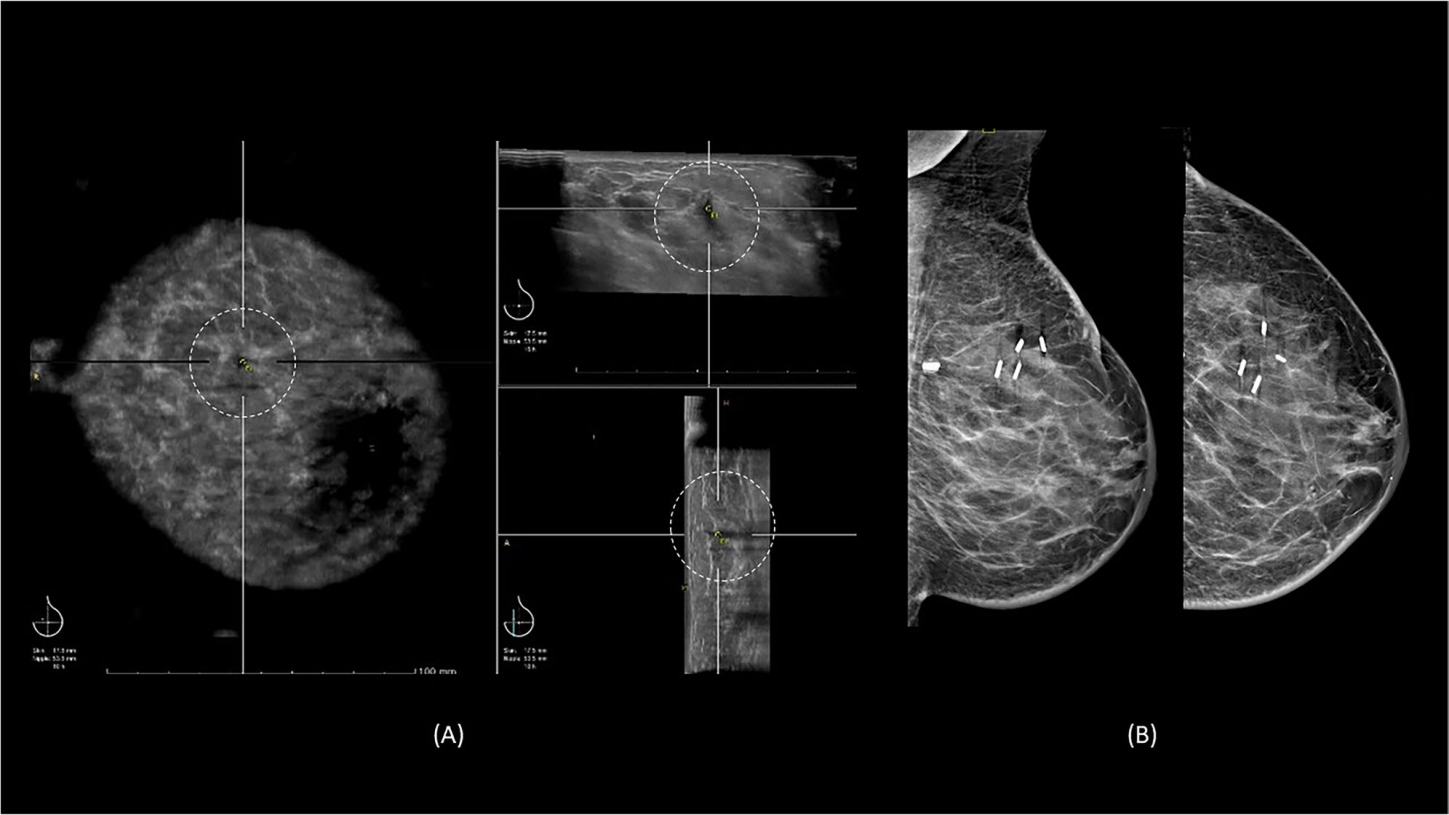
A 60-year-old woman with a history of breast cancer in the left breast. A There is an 8-mm lesion with architectural distortion in the left breast upper inner quadrant on 3D-ABUS (dashed circle). B Mammography of the left breast noted BI-RADS density C with surgical clips from previous surgery and no suspicious lesion was detected. Ultrasound-guided biopsy of the lesion confirmed invasive carcinoma NST, oestrogen receptor positive (50% positive), progesterone receptor, and HER-2 negative
